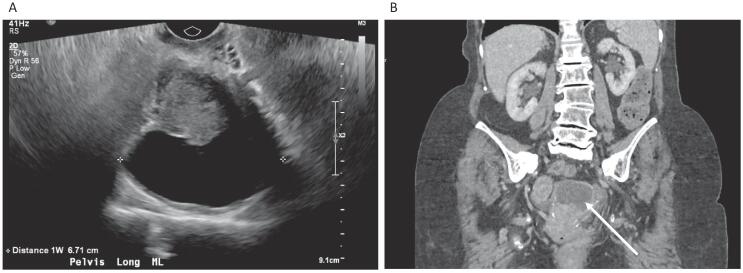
Pre-operative imaging. Legend. (A) Pelvic ultrasound demonstrating transverse image of the uterus, with cystic lesion at the uterine fundus measuring over 6 cm in greatest dimension, (B) CT abdomen/pelvis demonstrating pelvic cystic lesion (arrow).
